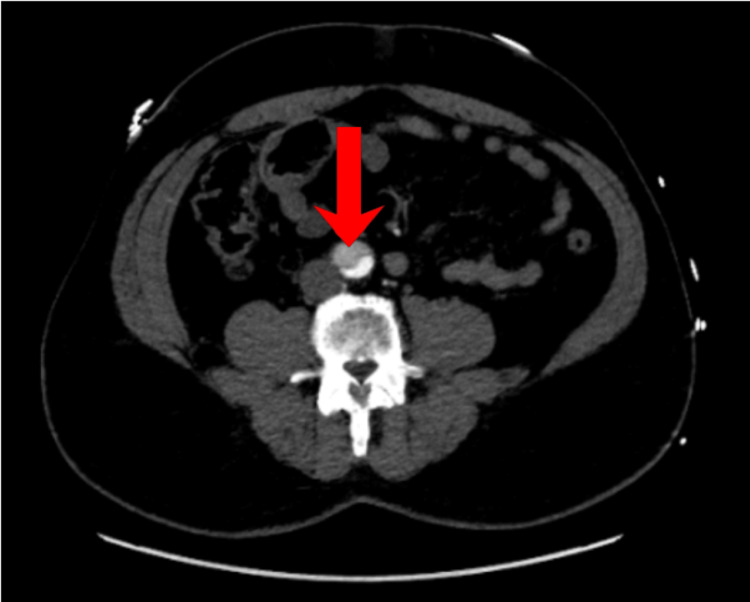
CTA chest axial view of aortic dissection (false lumen in abdominal aorta, red arrow) CTA: computed tomography angiography
